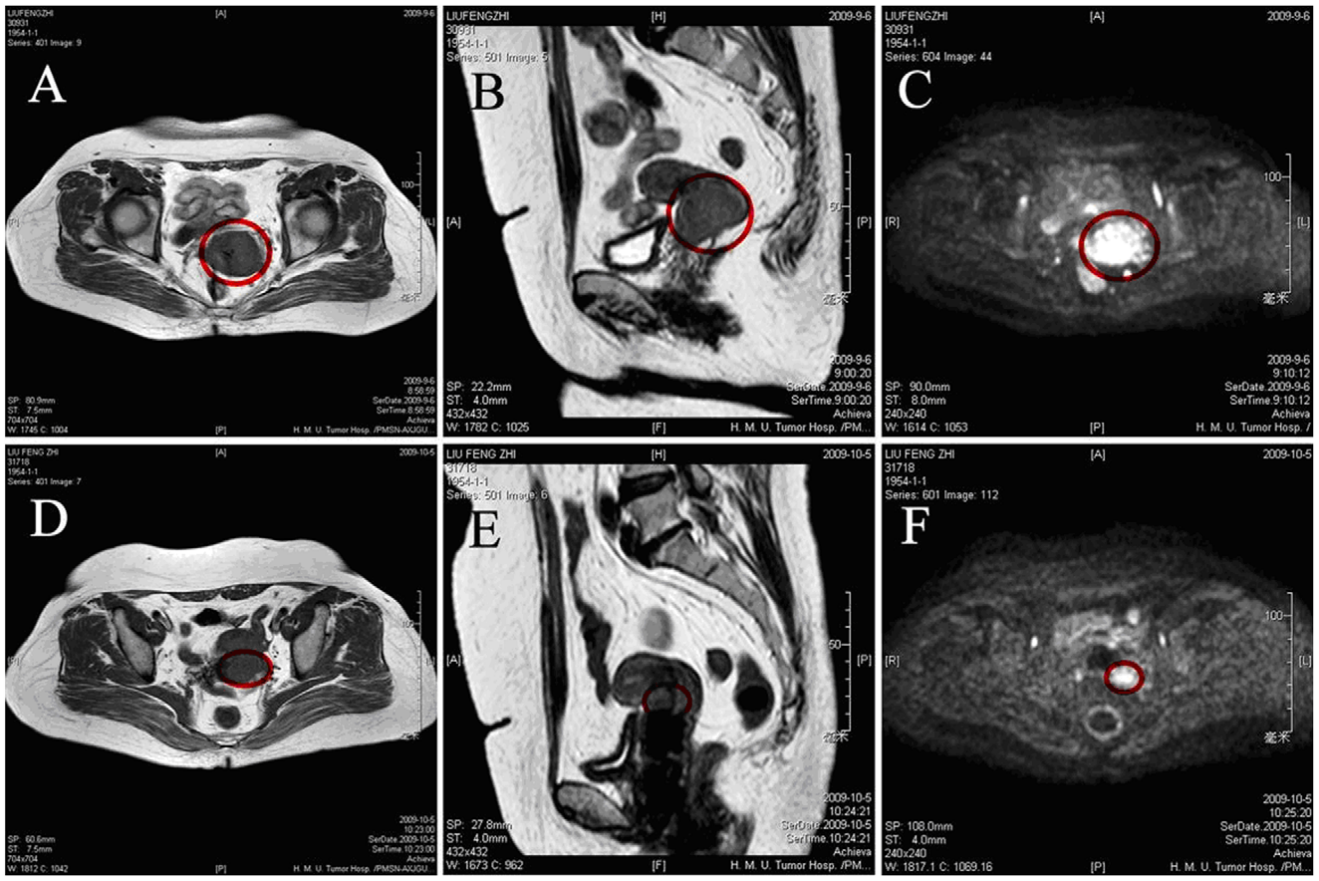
Conventional and DW-MRI of the same lesion from a 55-year-old woman undergoing NACT. (A)–(C): pretreatment axial (A) and sagittal (B) conventional MR images and diffusion-weighted MR image (C). (D)–(F): preoperative axial (D) and sagittal (E) conventional MR images and diffusion-weighted MR image (F). The red circles in (B)–(F) indicate the largest pretreatment and preoperative lesion as measured in different planes and using different MRI techniques.
